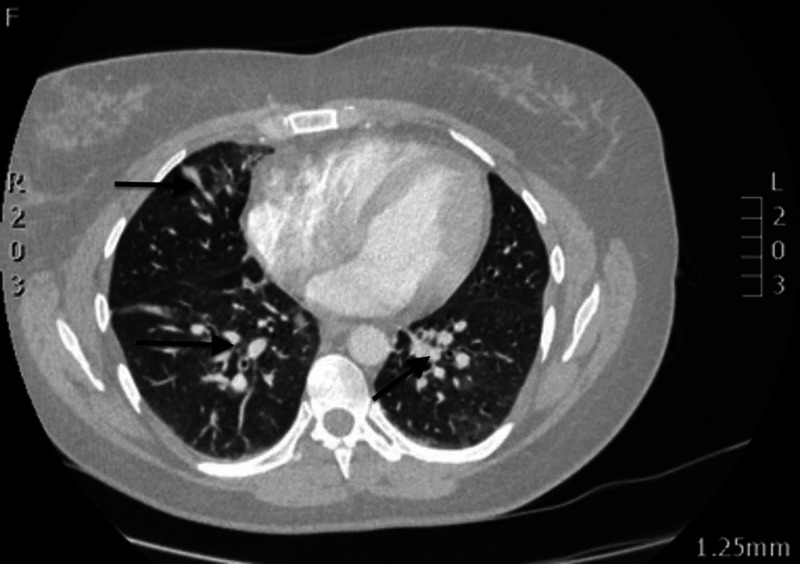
CTPA image (24/09/2020) showing multi-lobar pneumonia changes in the middle zones of both lungs (black arrows) CTPA, computed tomography pulmonary angiogram
